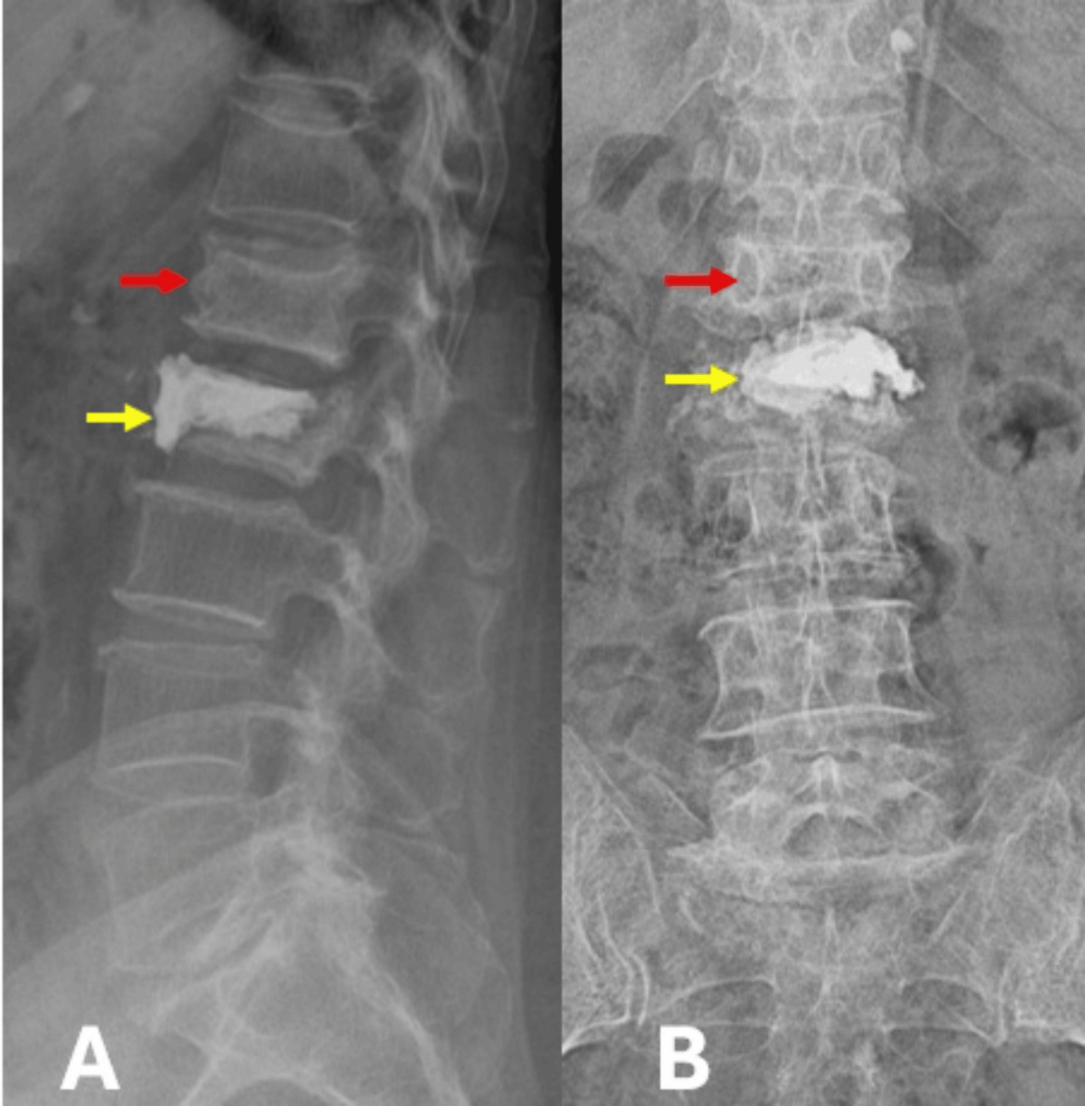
(A) Post-vertebroplasty lateral-view plain radiograph of the lumbar spine showing polymethylmethacrylate cement within the L2 vertebral body (yellow arrow) and a new wedge compression fracture of the adjacent L1 vertebra (red arrow). (B) Anteroposterior view confirming cement deposition in the L2 vertebral body (yellow arrow) with adjacent-level vertebral collapse at L1 (red arrow).
